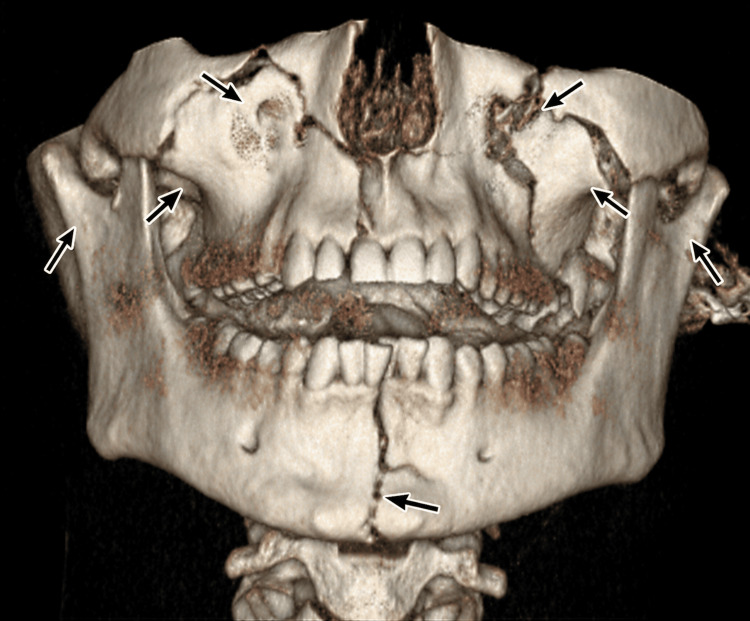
3D reconstruction of CT head and facial bones demonstrating a mandibular symphyseal fracture with a comminuted Le Fort II fracture pattern, as well as bilateral superolateral TMJ dislocation. The lateral arrows indicate the bilaterally displaced condylar fragments consistent with superolateral TMJ dislocation; the upper and midfacial arrows indicate the Le Fort II fracture lines; and the inferior arrow indicates the mandibular symphyseal fracture line. CT, computed tomography; TMJ, temporomandibular joint
